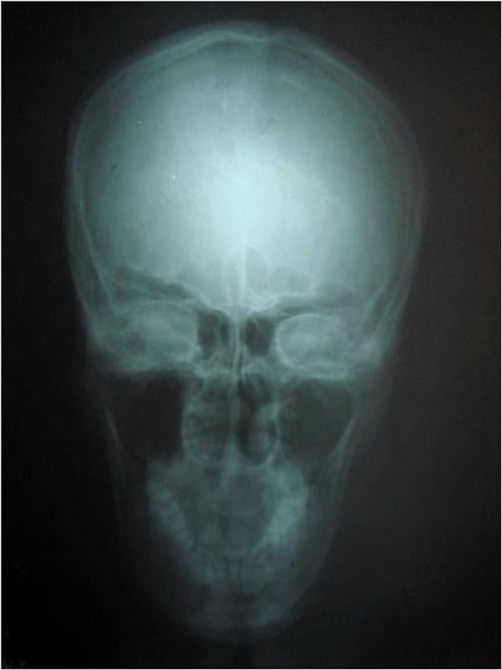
Photograph of the X-ray skull showing both sides equal.
